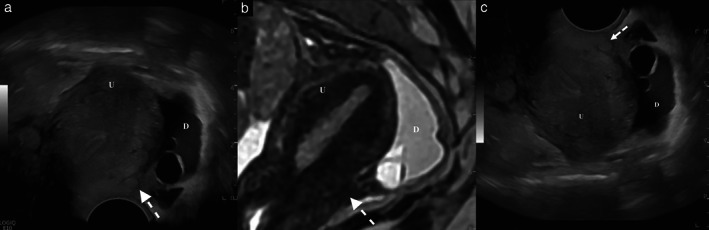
Fusion imaging in a patient with superficial peritoneal and retrocervical endometriotic lesions. Corresponding sagittal planes obtained by transvaginal sonography (TVS) (a) and magnetic resonance imaging (MRI) (b), with additional reconstructed top‐down TVS image (c), showing the uterus (U) and the pouch of Douglas (D). In both modalities, the presence of pelvic fluid containing cystic hemorrhagic peritoneal lesions and deep retrocervical endometriosis can be seen, which is echogenic on TVS and hypointense on T2‐weighted MRI (arrows).
